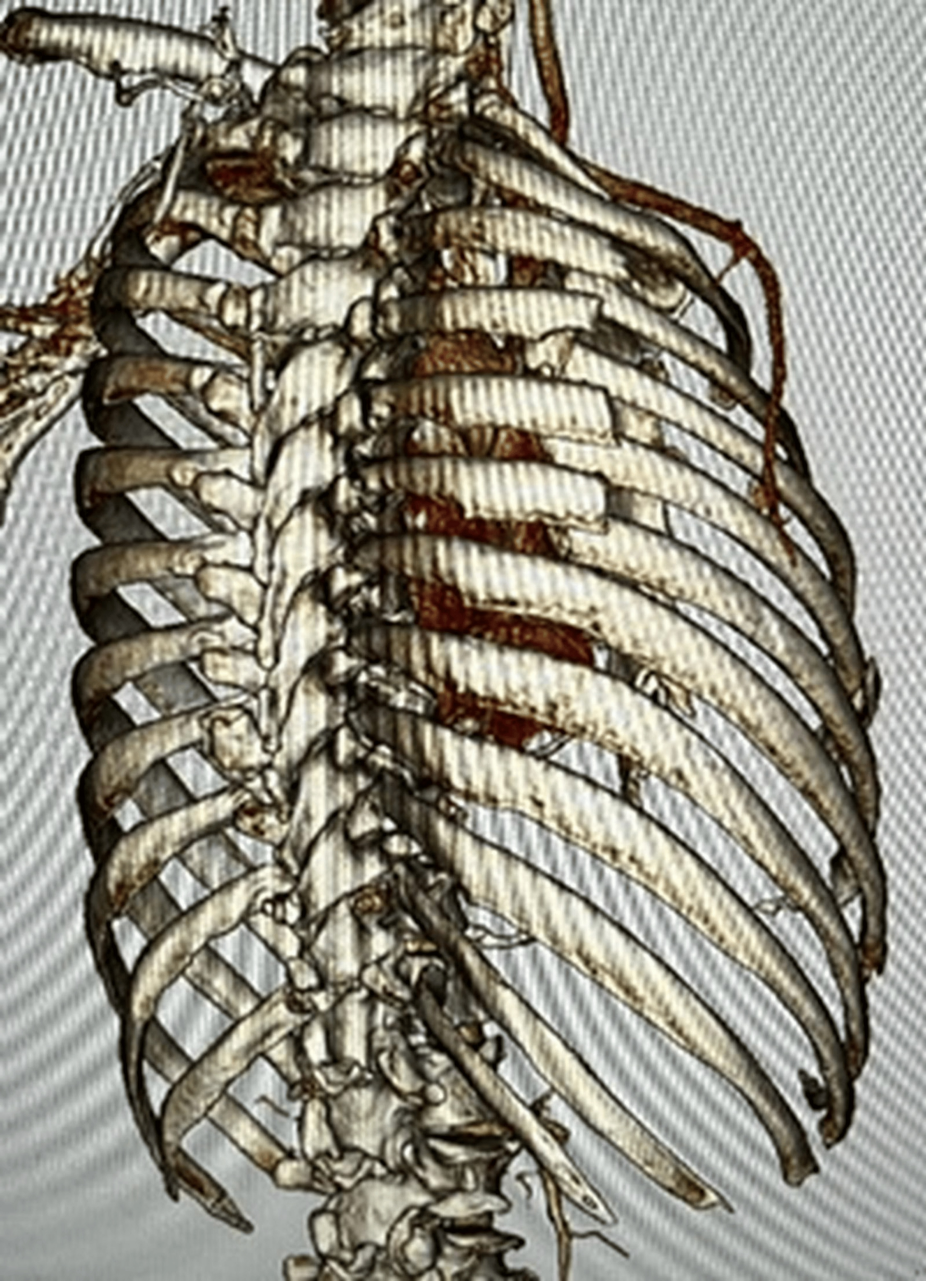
3D CT image on admission - 2 A fracture of the right scapular body was present, with multiple rib fractures accompanied by a flail segment just below it CT: computed tomography
